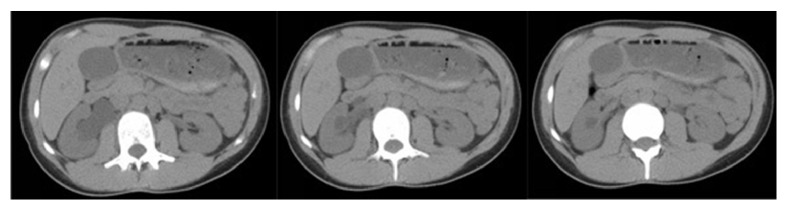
Noncontrast CT scan of the abdomen with moderate right-sided hydronephrosis proximal to an apparent lower pole renal vessel overlying the ureteropelvic junction performed at the time of acute pain.
